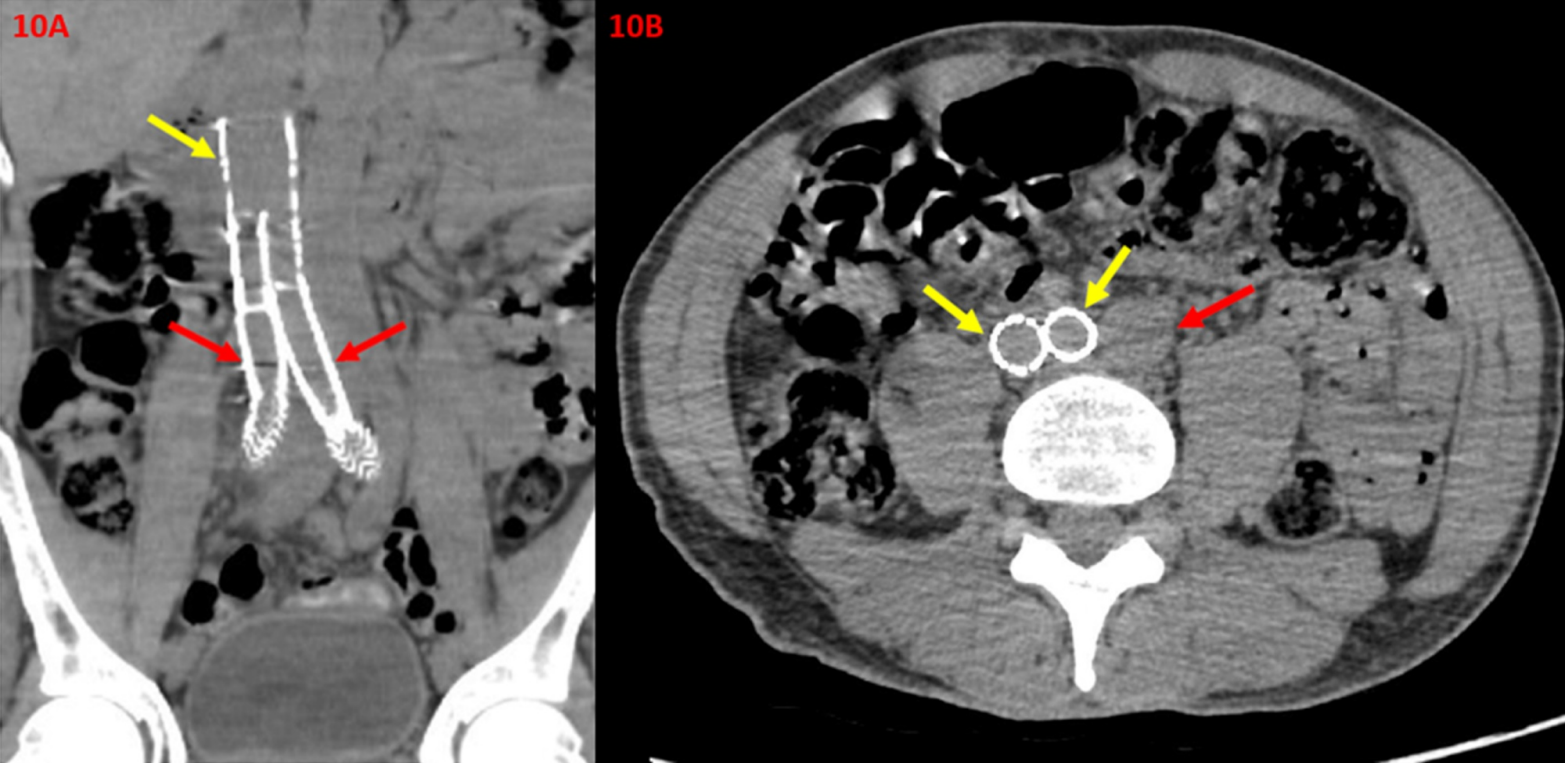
One-month Post-operative Non-contrast Enhanced Computed Tomography Images Displaying Stent Graft Patency within the Iliocaval Confluence A one-month post-operative non-contrast enhance coronal computed tomography of the abdomen and pelvis (10A) demonstrates patency within the iliocaval confluence (yellow arrow) and right and left iliac veins (red arrows). There is no evidence of thrombus, significant stenosis, or graft failure. A one-month post-operative non-contrast enhance axial computed tomography of the abdomen and pelvis (10B) demonstrates patency of the right and left iliac veins (yellow arrows) with no evidence of thrombus. Additionally, the unremarkable aorta (red arrow) demonstrates patency with no evidence of aneurysmal dilation or abnormality.
